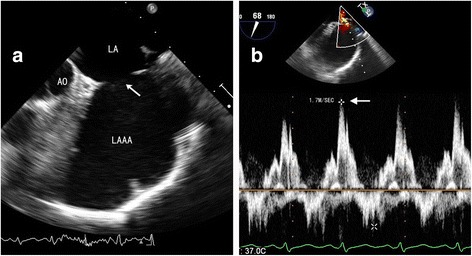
Details of the aneurysm on transesophageal echocardiography. a A clear orifice (*arrow*) is present between the aneurysm and the LA. b Pulsed wave Doppler imaging showing blood flow at the orifice. Flow velocity was approximately 1.7 m/s
